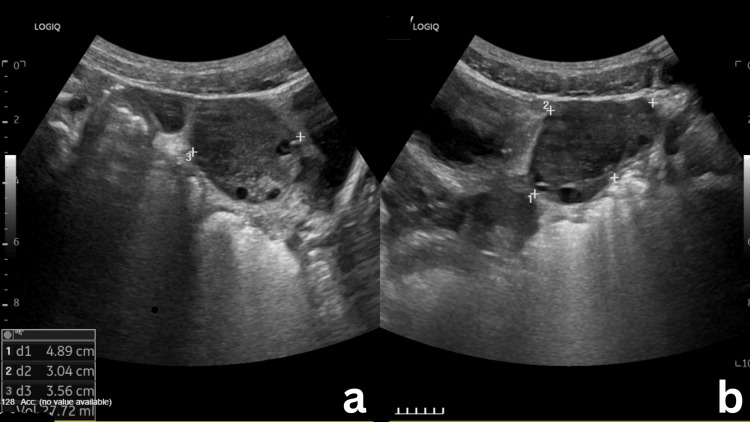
Ultrasound findings of the left ovary (a) Enlarged ovary with stromal edema and peripheral displacement of follicles. (b) Ultrasound measurements demonstrating increased ovarian dimensions and an ovarian volume of 26.72 mL.
